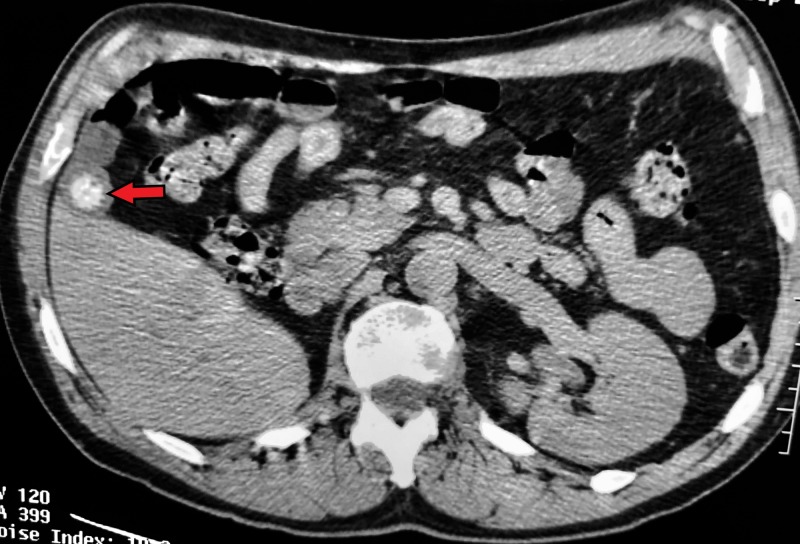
Axial CT of the abdomen without contrast taken 10 years prior. Prominent irregular calcification visible in the gallbladder fundus (arrow) CT - Computed tomography
